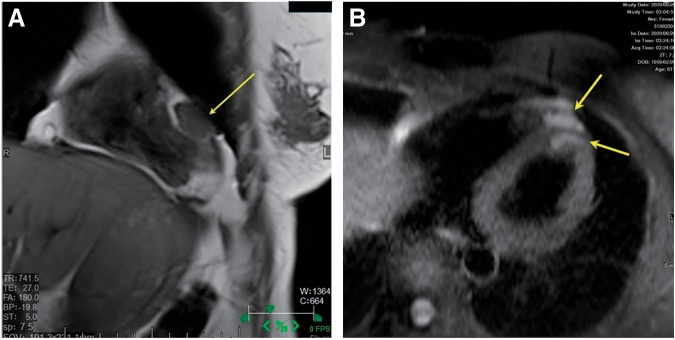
Cardiac MRI images clearly detected the cardiac metastatic lesion with intermediate intensity on T1-weighted images (A) and high intensity on T2-weighted fat-suppression images (B) (cardiac tumor: yellow arrows).
